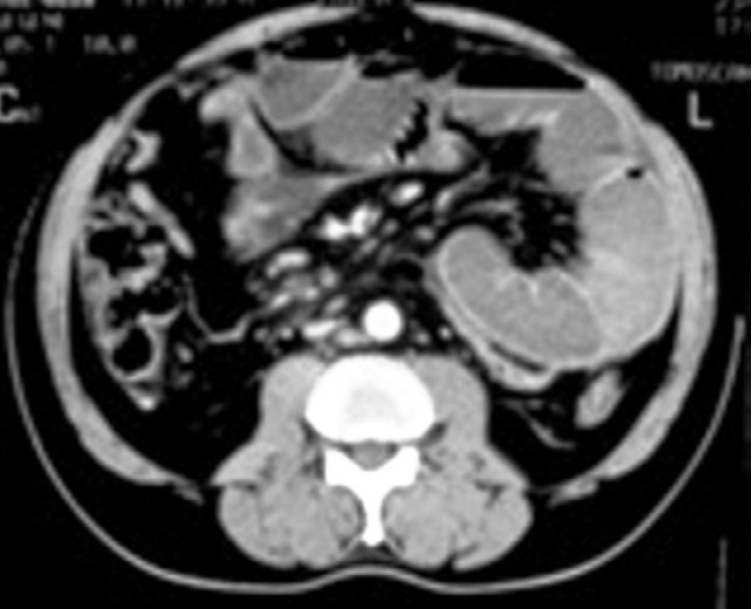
Abdominal CT showing small bowel obstruction.
